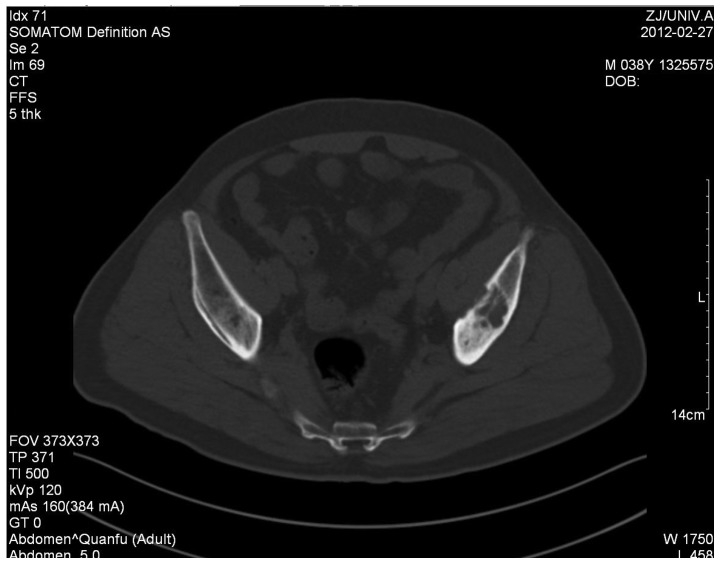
Computed tomgraphy (CT) scan of the pelvis demonstrating an osteolytic lesion in the left ilium.
